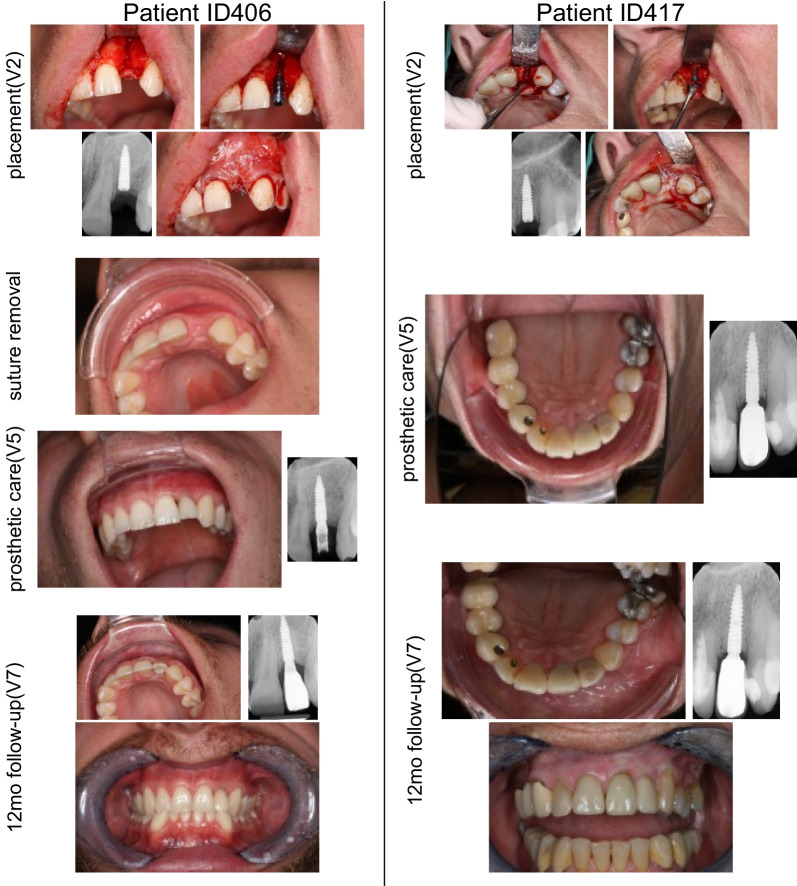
Surgical, follow-up and X-ray images of the representative clinical cases. Clinical and X-ray pictures were taken during implant placement, suture removal, prosthetic care, and 12-month follow-up for 2 representative patients
